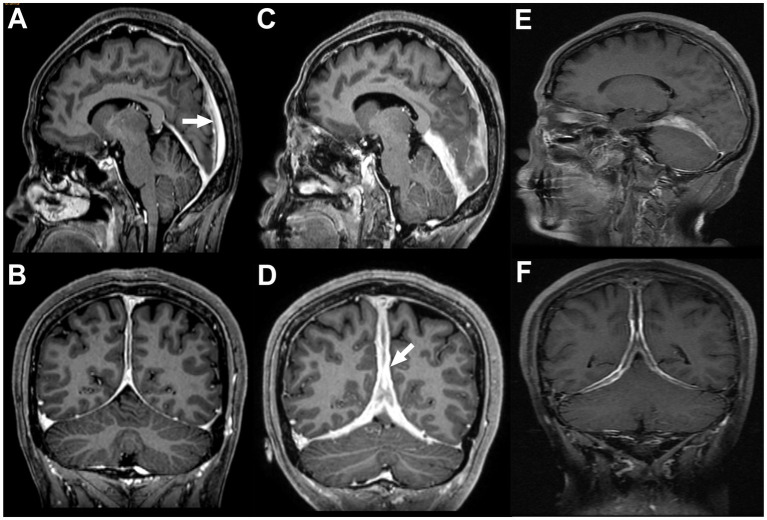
Contrast-enhanced sagittal (A,C,E) and coronal (B,D,F) T1-weighted images obtained on May 24, 2021 (A,B), April 22, 2022 (C,D), and October 11, 2023 (E,F). White arrows indicate areas of dural thickening and contrast enhancement. Compared with earlier examinations, both the extent and intensity of enhancement were substantially reduced on October 11, 2023.
